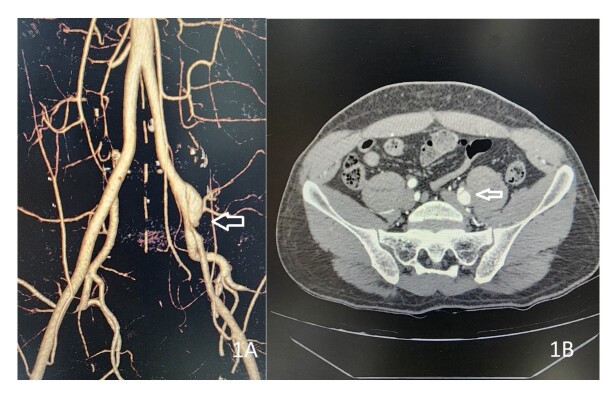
(A) Reconstructed CT image depicting the narrow true lumen of the EIA; (B) Axial CT image depicting the origin of the dissection.
